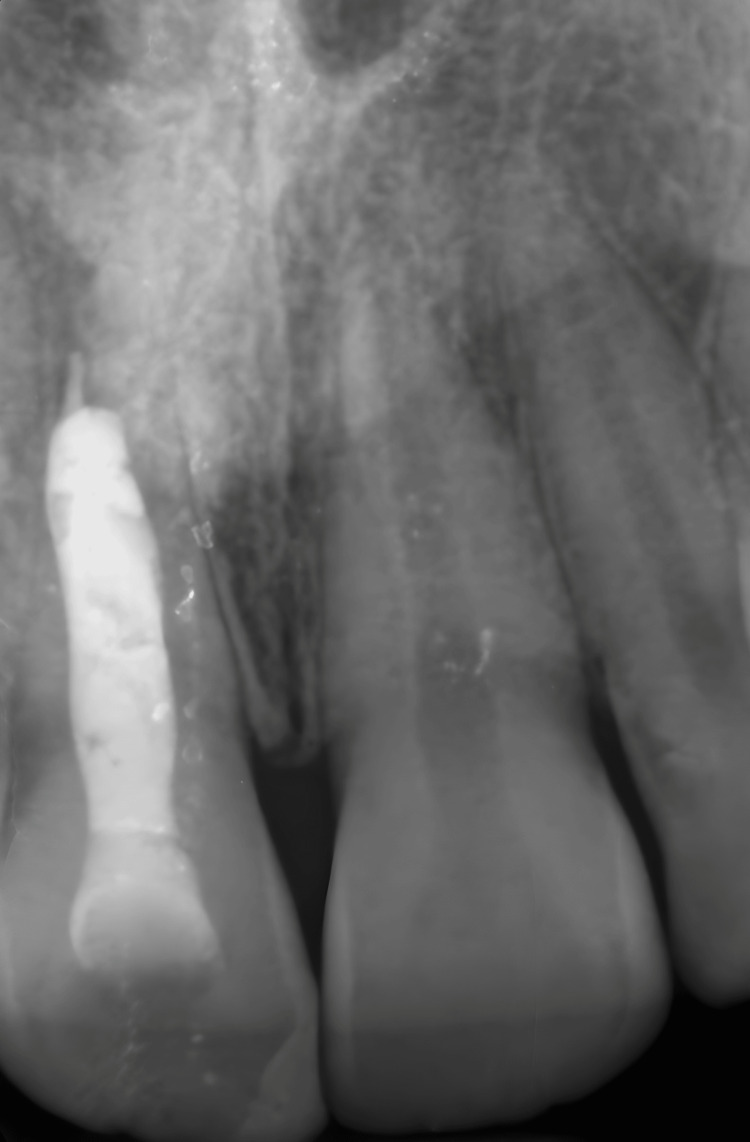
Final X-ray after endondontic treatment of the right central incisor The apical third root filling was with Super EBA™ (Harry J. Bosworth Co., Skokie, IL) and warm guttapercha was used for the backfilling.
